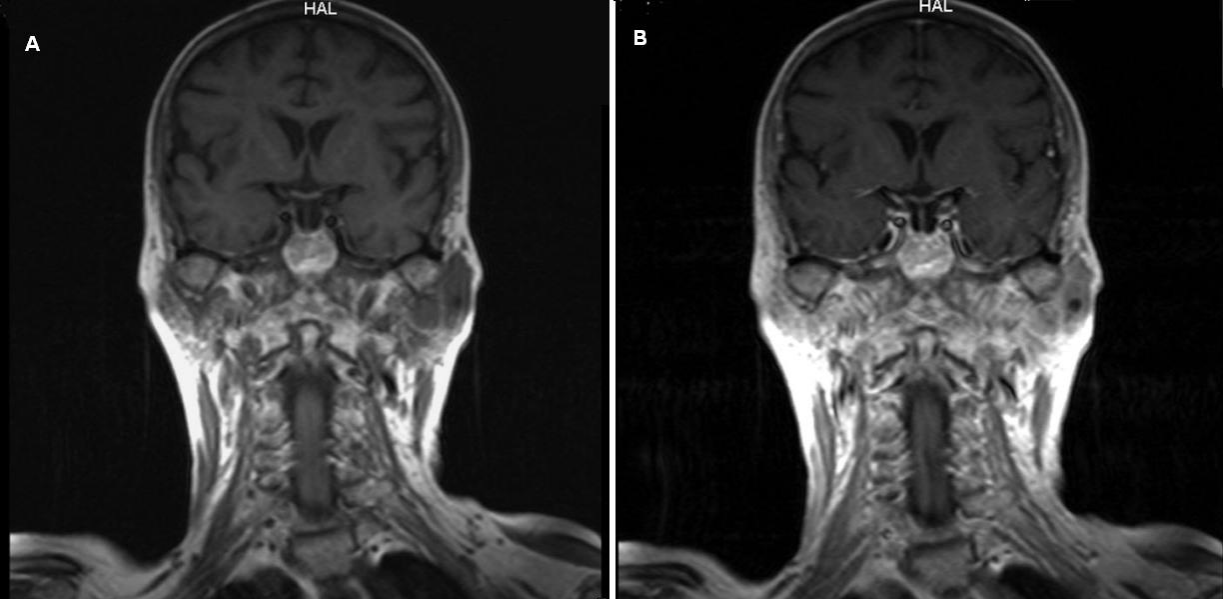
(A) Coronal T1-weighted image shows the inhomogenic appearance of the left parotid gland. (B) Coronal T1-weighted image after gadolinium contrast injection shows mild enhancement of the solid components, while the cystic areas remain hypodense.
